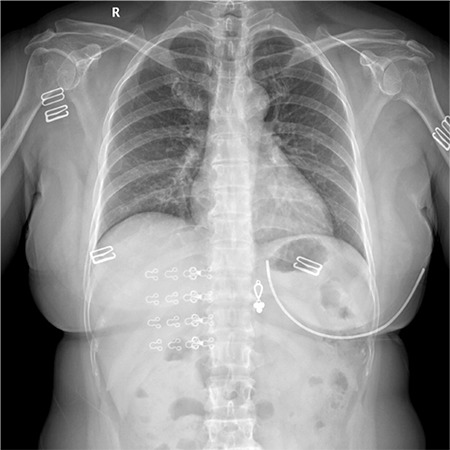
Initial admission chest X-ray shows increased and thickened right lower lung markings, suggesting bronchitis and interstitial pneumonia.
